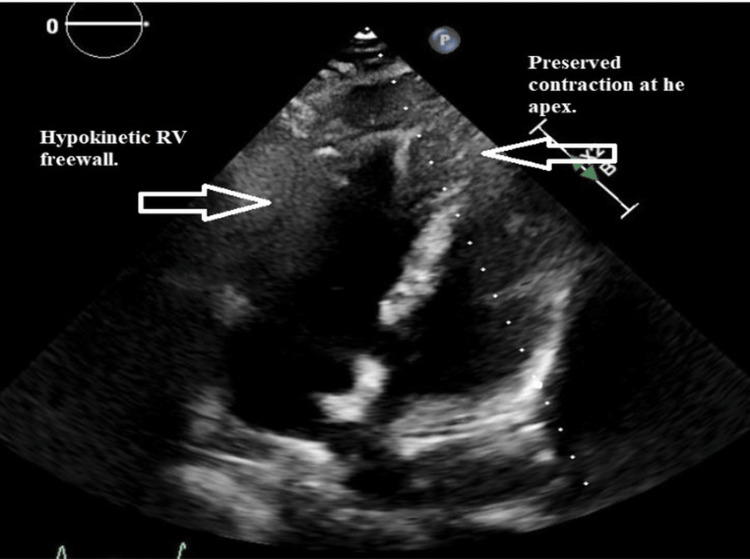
Apical four-chamber transthoracic echocardiogram demonstrating a severely dilated right ventricle (RV) with preserved apical contractility (McConnell’s sign; arrows).
